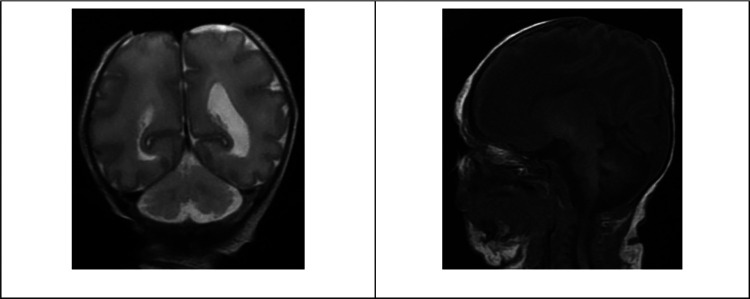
Patient head MRI. Selective images of the MRI coronal T2 and sagittal T1-weighted images showing the absence of scalp and calvarium in the parietal region with no herniation.
